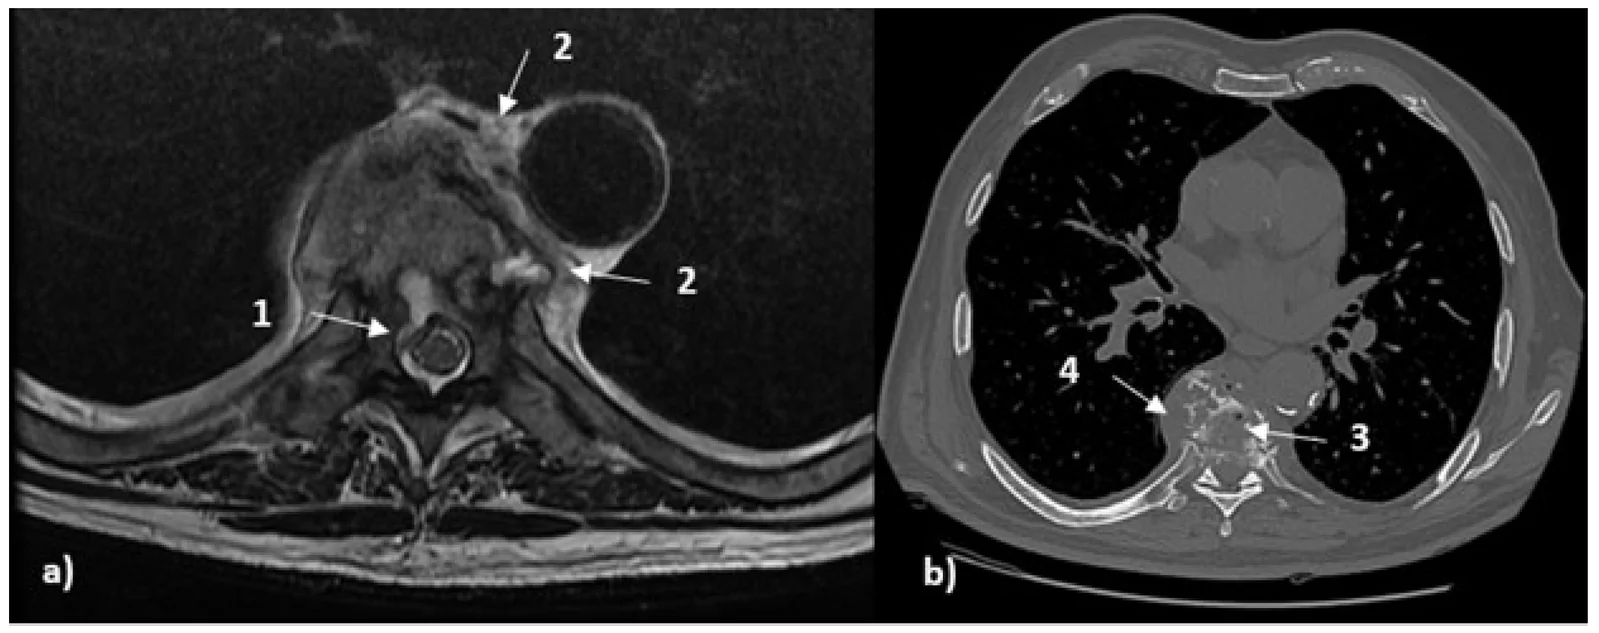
Tuberculous pattern on MRI and CT: body-centred destruction with thin-walled paravertebral extension. Axial T2-weighted MRI (a) and axial CT (b) at D8 show heterogeneous vertebral body involvement, epidural abscess with dural indentation (1), and sharply marginated paravertebral collections (2, 4) with associated osseous destruction (3)—an imaging constellation that shifts probability towards TS rather than ordinary PS.
